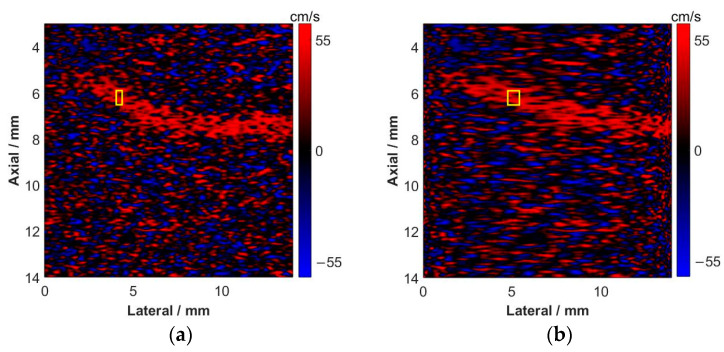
Flow image information calculated with color flow Doppler: (a) original image without motion compensation and (b) the compensated image. The yellow rectangular area was used to measure the average blood velocity.
